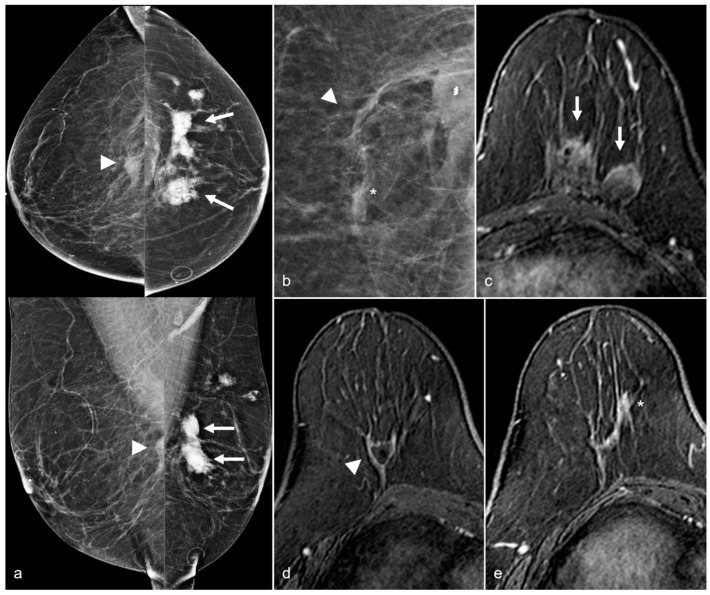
A 65-year-old woman with a history of a ruptured subglandular silicone implant. Screening mammogram (a) demonstrates very dense masses (arrows) in the left prepectoral region on the CC and the MLO views. Suspicious right breast calcifications (arrowhead) were biopsied with a biopsy clip seen in a hematoma in the right prepectoral region on the CC and MLO views. Pathology revealed ductal carcinoma in-situ. An additional biopsy was performed in the right breast for a small focal asymmetry (*) better seen on magnified CC view (b) and pathology demonstrated invasive ductal carcinoma. MRI demonstrates a T1 isointense peripherally enhancing silicone granuloma in the left breast prepectoral region ((c), arrows). In the right breast, a biopsy clip is seen within the biopsy cavity ((d), arrowhead). Anterior and inferior to this region (e), there is a linear non-mass enhancement. More anterior and medial to the non-mass enhancement, there is an enhancing mass (*), corresponding to the biopsy-proven invasive ductal carcinoma.
